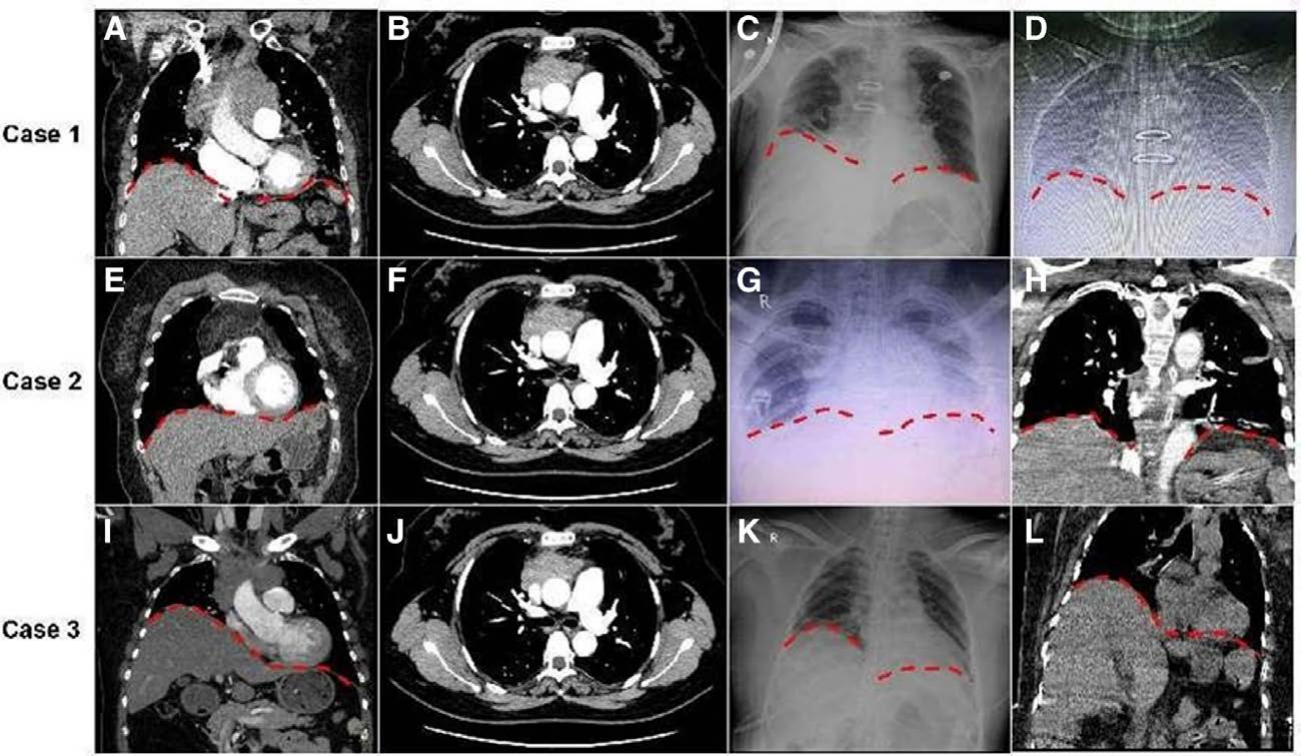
Radiological features of patients with massive mediastinal tumors before and after surgery. (A, E, I) Coronal plane of chest CT before surgery, (B, F, G) Horizontal plane of chest CT before surgery, (C, G, K) Chest X-ray on postoperative day (POD) 2, (D) chest X-ray on POD 20, and (H, L) coronal plane of chest CT on POD 23. Red dotted line indicates diaphragm.
